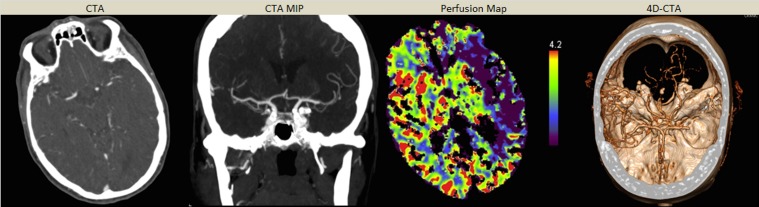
Reviewing example of a patient that presented to the ER with right-sided hemiplegia and dysarthria. A difficult to read CTA due venous enhancement just siding the right MCA was performed, and intra-cranial vessels where initially declared patent. Only when reviewing the 4D-CTA data, the patient was transferred to the angio-suite for IA therapy. Below figs shows consecutively CTA, coronal MIP view, perfusion map and a freeze of the 4D-CTA.
